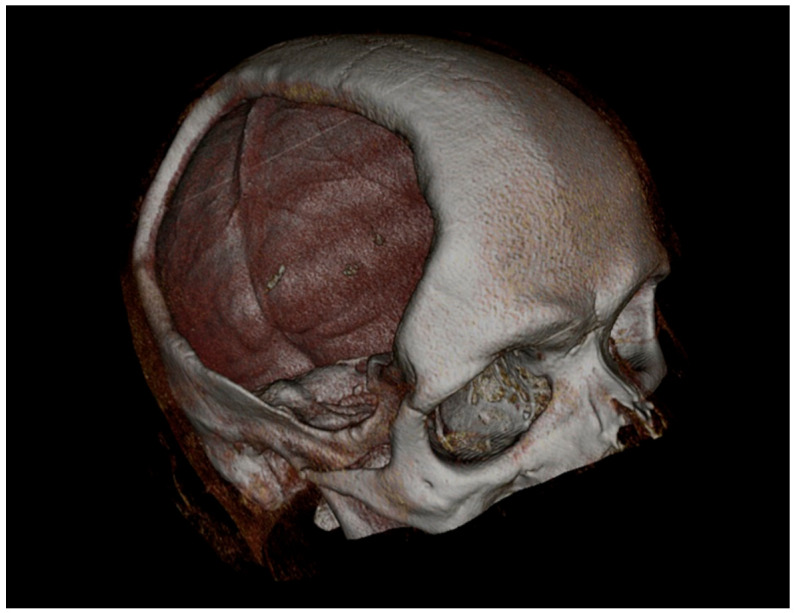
3D rendering of a non-contrast CT head performed for cranioplasty designing purposes. At the Oxford Cranioplasty Pathway all calvarial defects secondary to decompressive craniectomies are studied with CT images acquired using a dedicated cranioplasty protocol (Siemens SOMATOM Drive): 120 kV and 300 mAs with automated tube current modulation (CARE Dose4D). The 3D models are generated from 0.5 to 0.7 mm thin-slice reconstructions to accurately visualize the bony defect (Kernel Hr60) and surrounding soft tissue contours (Kernel Hr38), whereas brain imaging is obtained with conventional 5 mm thin-slice reconstructions (Kernel Hr38).
